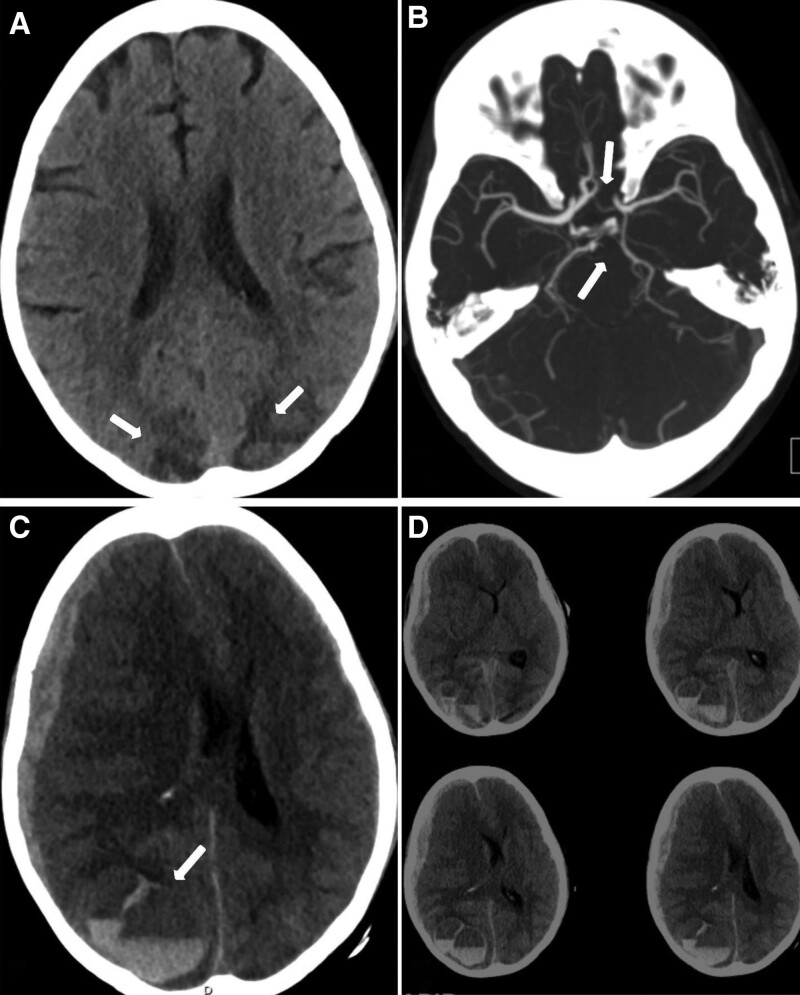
(A) Initial CT scan of the head demonstrating bilateral parieto-occipital hypodensities with mixed hyperdensity signal concerning for PRES. (B) CT angiogram axial view of the circle of Willis. Top arrow depicting an aplastic A_1_ segment of the left anterior cerebral artery (ACA). Bottom arrow showing a fetal origin of the left posterior cerebral artery (fPCA). (C) Repeat CT scan axial view of the head 1-hour after the initial scan showing significant fluid-fluid levels (arrow) representative of thrombocytopenia related intraparenchymal hemorrhage in PRES. Additionally, a 15 mm leftward midline shift, bilateral subdural hematomas, and right uncal herniation. (D) Multi-slice images detecting intracerebral hemorrhage. CT = computerized tomography, PRES = posterior reversible encephalopathy syndrome.
